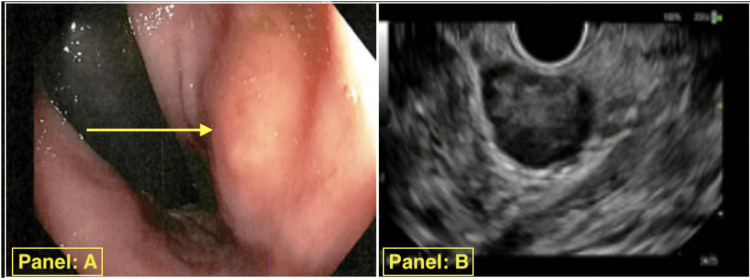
Routine colonoscopy revealed a 25 mm x 13 mm x 13 mm submucosal rectal mass (left panel A), confirmed by endoscopic ultrasound as a hypoechoic, heterogeneous lesion originating from the muscularis propria (right panel B)
